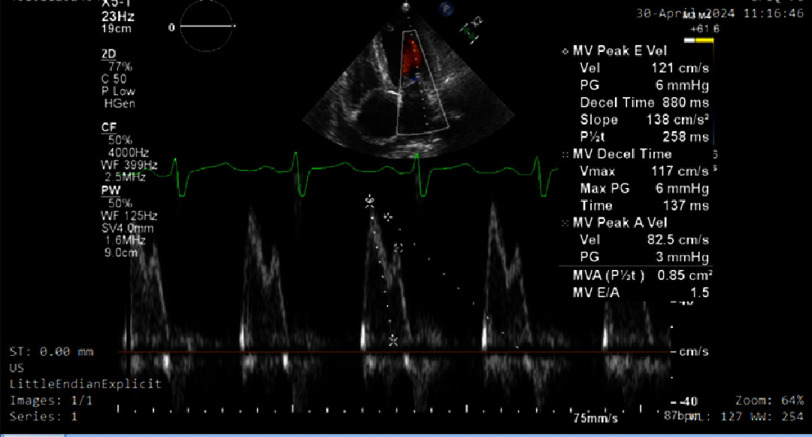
Pulsed wave Doppler recording across the mitral valve demonstrating peak early (E) diastolic velocity of 121 cm/s and late (A) diastolic velocity of 82.5 cm/s, yielding an E/A ratio of 1.5. This pseudonormalized filling pattern reflects elevated left ventricular filling pressures despite relatively preserved E and A velocities, a common finding in cardiac amyloidosis.
